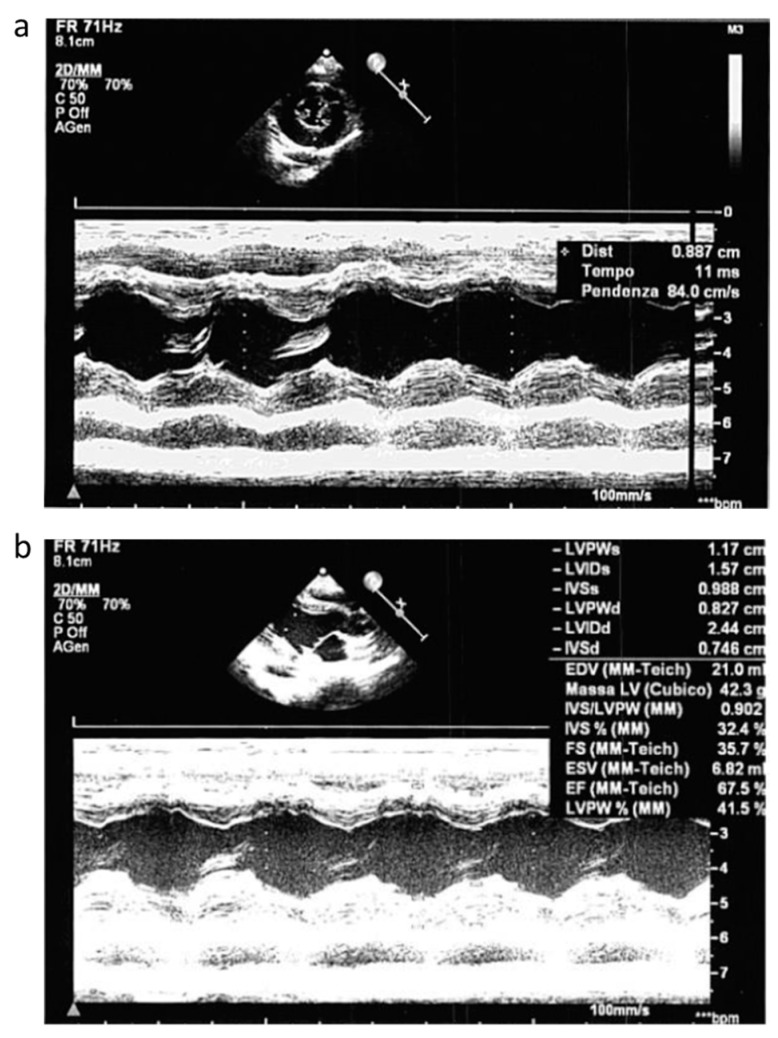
Echocardiogram pattern. Echocardiogram performed during acute phase that showed moderate hypertrofic cardiomyopathy of the left ventricle (a) without outflow obstruction and with normal EF (b).
